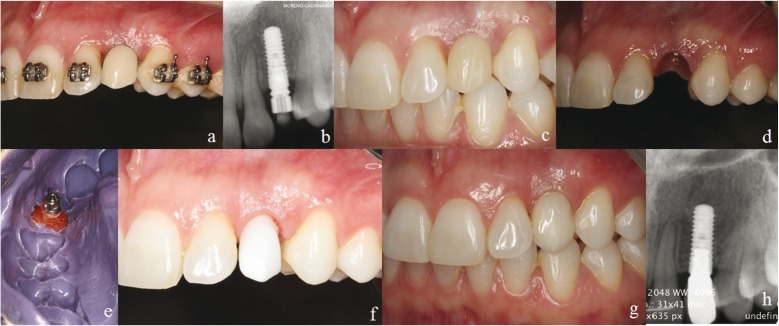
Restorative procedure. a) Screw-retained provisional acrylic crowns was used to performe the peri-implant soft tissue conditioning phase. Note the total absecnce of mesial papilla. Around 2 to 3 mm of root cementum of the distal aspect of the left upper lateral incisor is clinically visible. b) Periapical radiographs of the implant loaded. Note the correct level of the interproximal bone at the adjacent teeth. c) After 6 month, complete papilla fill was recorded. d) Emegence profile the day of the final impressions. Note the scalloped image. e) Customized impression of the implant and the sof tissue. f) Zirconia structure design CAD/CAM technology. g) Final direct screw rehabilitation was inserted. Note the papilla fill. h) Periapical radiographs with the final restoration. Interproximal bone level are manteined.
